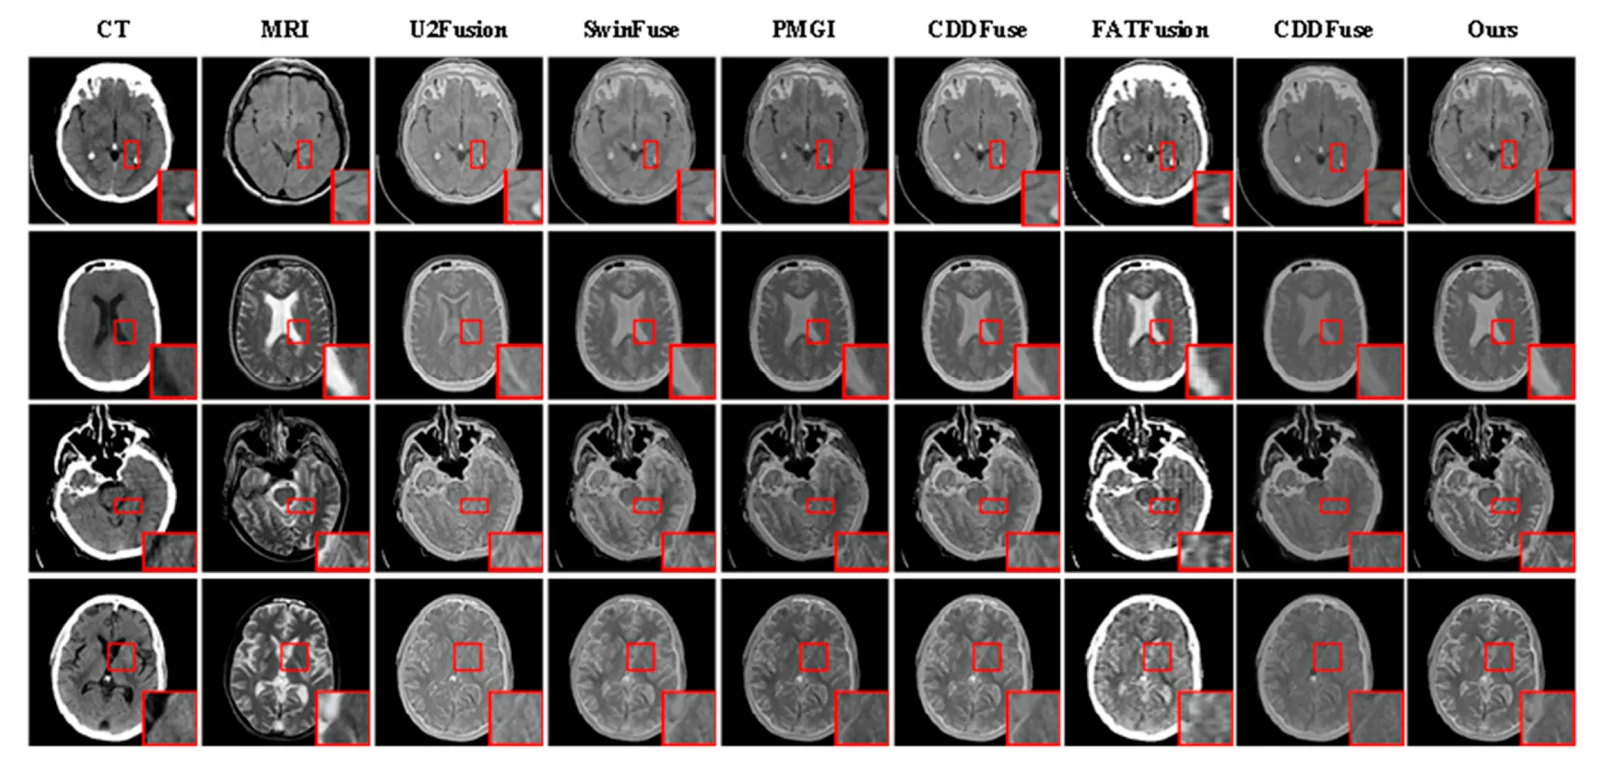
Qualitative comparison between the proposed method and six competing approaches on the CT-MRI dataset. Each row represents a different sample, and each column represents a different method.The red boxes denote the zoomed-in regions.
